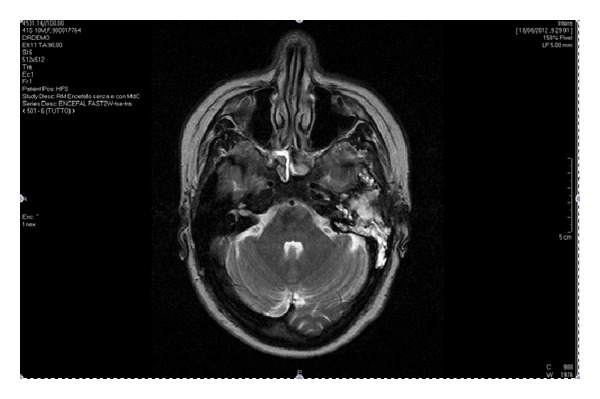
MRI revealing left mastoid cells and the middle ear occupied by a soft tissue density mass.
